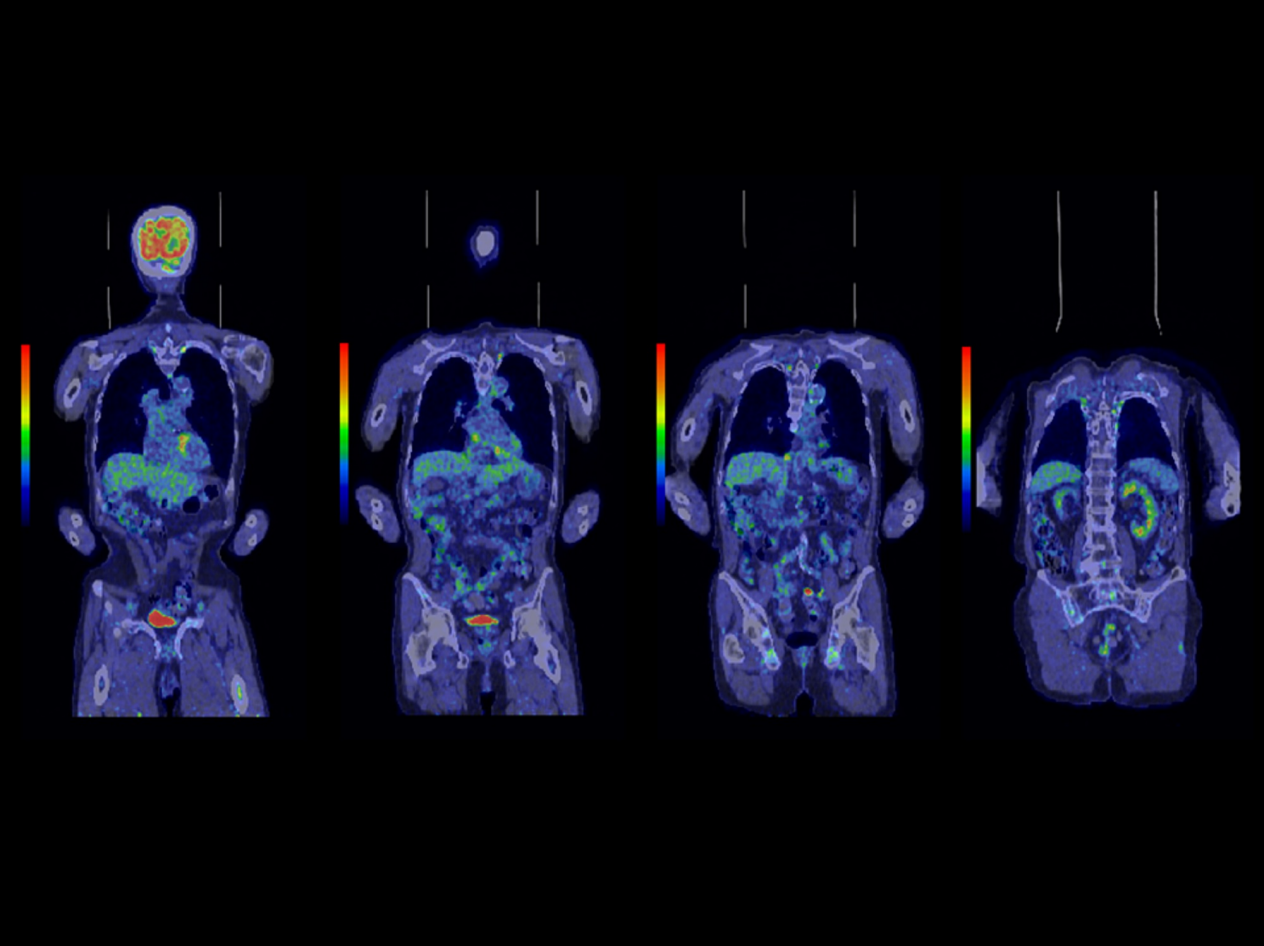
FDG-PET/CT scan three years and three months after initial treatment. Coronal sections from anterior to posterior are displayed from left to right. FDG uptake in IVC tumor and two lymph node metastases have disappeared. FDG-PET, fluorodeoxyglucose-positron emission tomography; IVC, inferior vena cava
